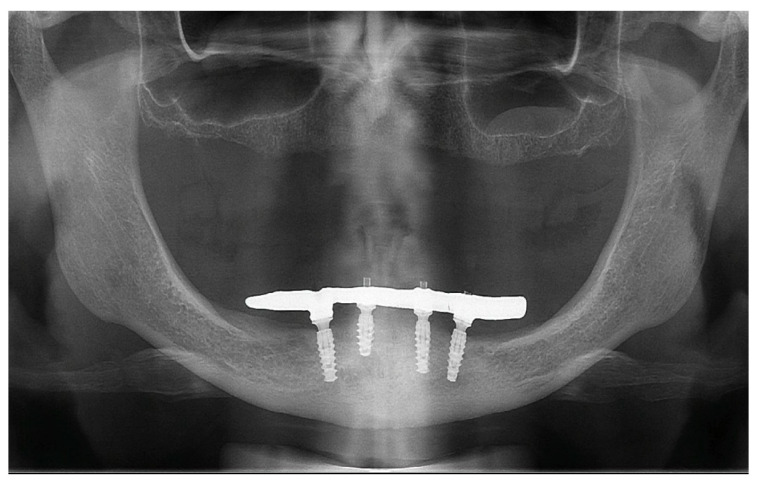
Panoramic radiograph at 24-month follow-up.
